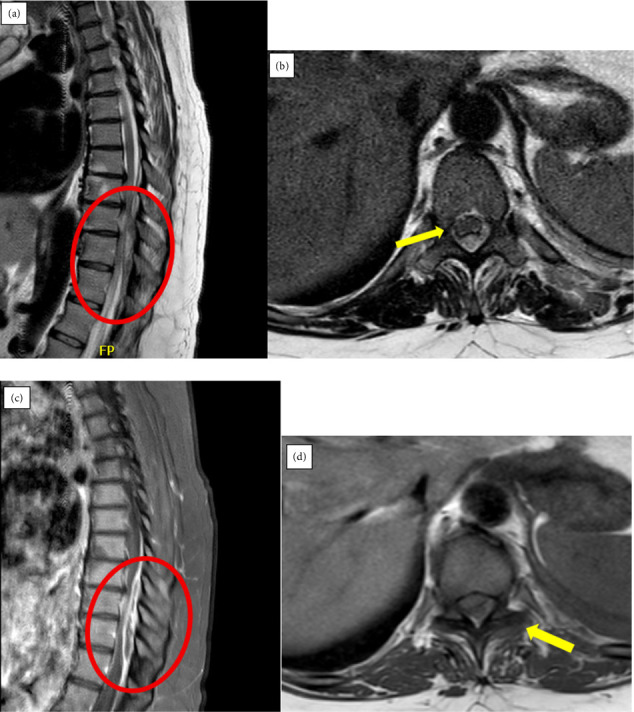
Sagittal (a) and axial (b) T2-weighted MRI images show a dorsal epidural fluid collection (yellow arrow) extending from T8 to T11 (red circle), consistent with an abscess, causing severe thecal sac effacement. Sagittal (c) and axial (d) postcontrast T1-weighted MRI images demonstrate abnormal enhancement in the left T9-T10 facet joint (yellow arrow), with associated effusion, paraspinal extension, and possible osseous erosion (red circle) findings consistent with septic facet arthritis.
